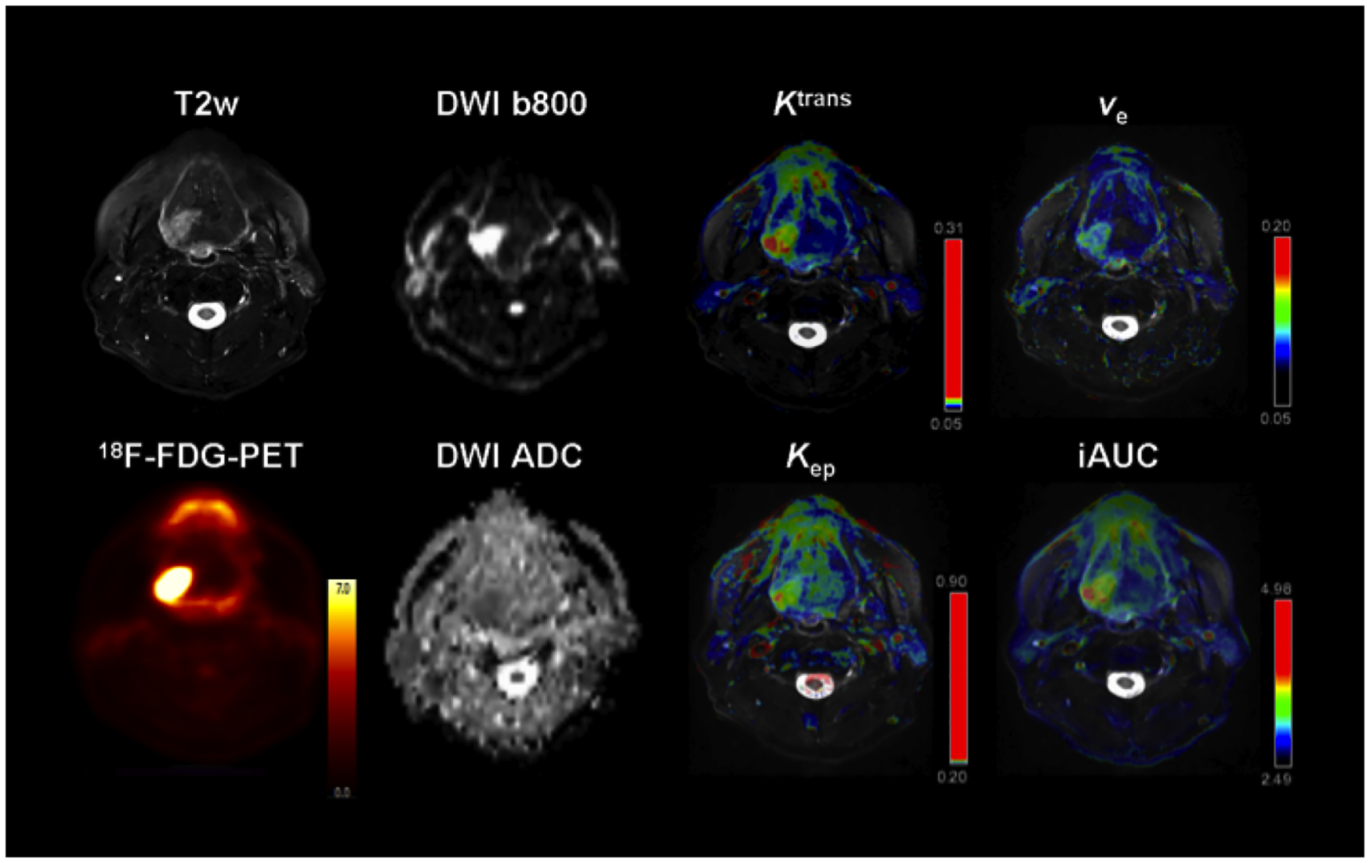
Example of a 59-year old male patient depicting the different molecular parameters obtained by simultaneous PET/MRI. Note the biopsy-proven secondary squamous cell carcinoma of the base of the tongue on the right side. 23 months ago the patient was operated on a squamous cell carcinoma of the soft palate on the same side.
